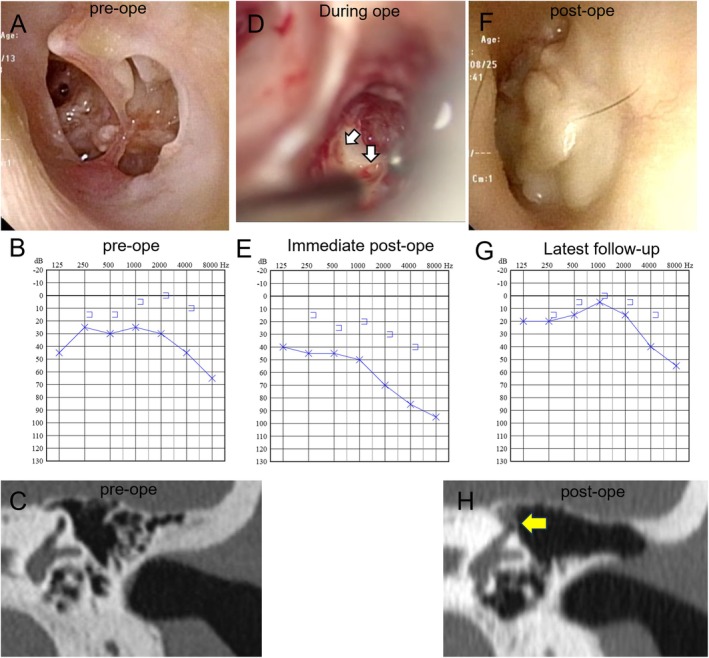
Case 2. (A) Preoperative tympanic membrane finding. (B) Preoperative audiometry. (C) Preoperative computed tomography findings. (D) Intraoperative findings. The white arrow indicates the bony defect in the posterior semicircular canal. (E) Postoperative audiometry after 2 weeks. (F) Postoperative tympanic membrane finding. (G) Postoperative audiometry after 2 years. (H) Postoperative computed tomography findings. The yellow arrow indicates the cartilage‐covered bony defect of the posterior semicircular canal.
